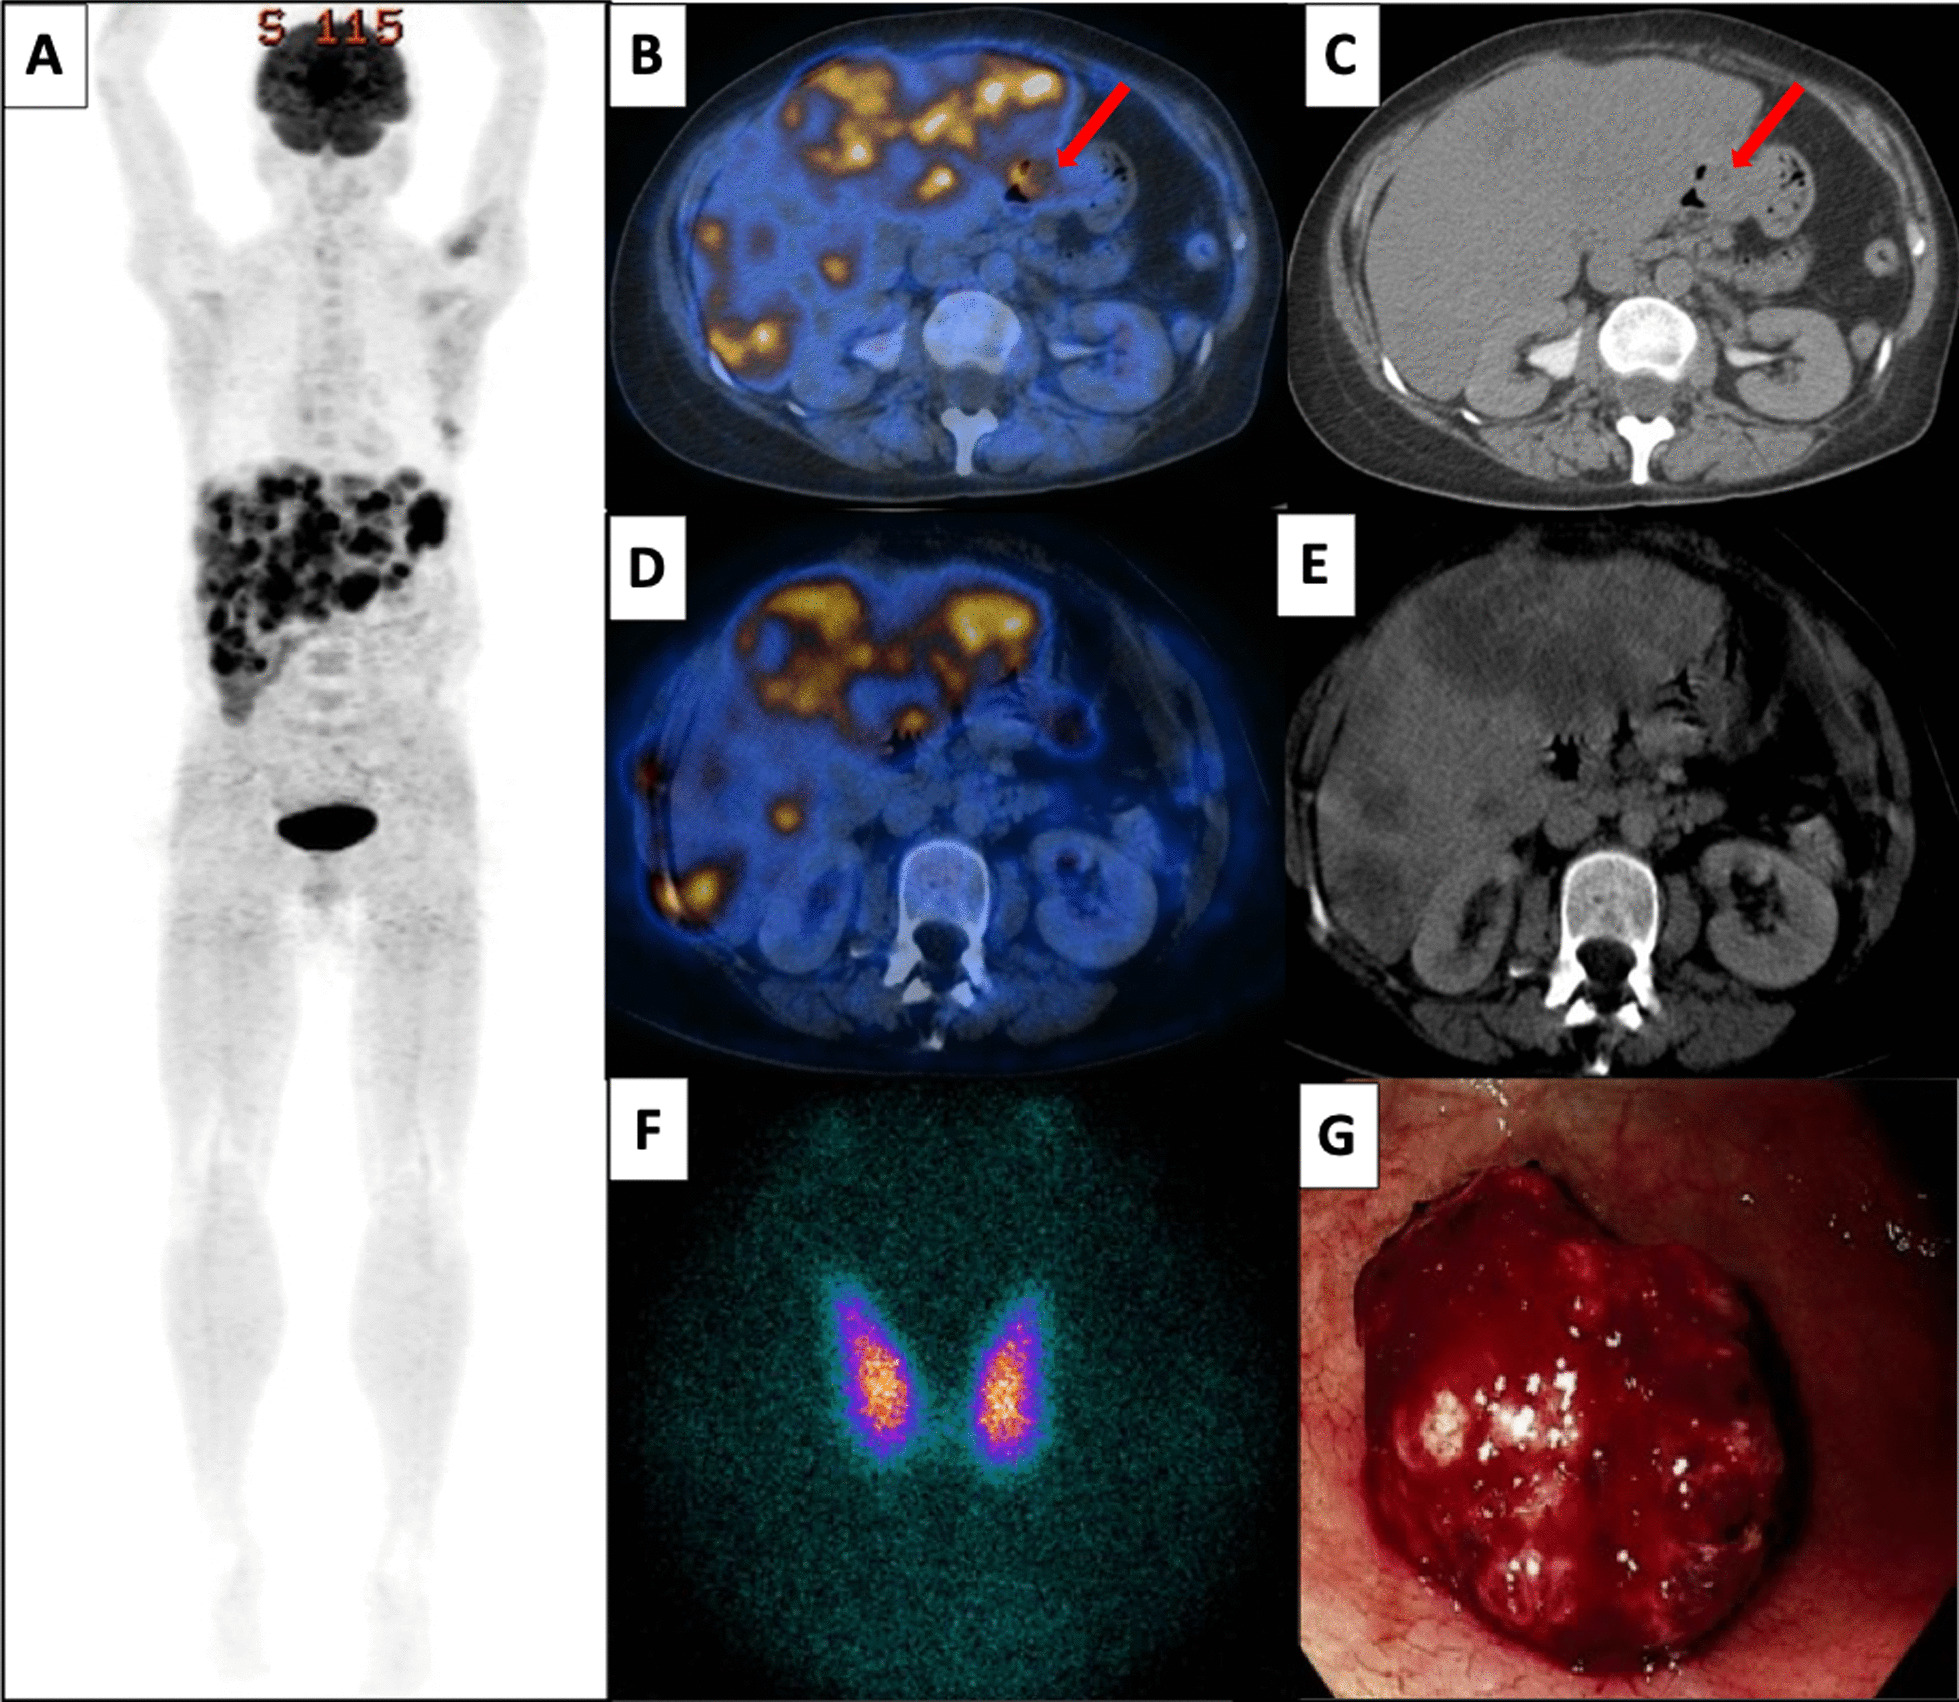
Imaging studies upon presentation and upper endoscopy. A–E Contrast-enhanced ^18^FDG PET-CT exhibited hepatomegaly (22 cm longitudinal axis) with multiple large FDG-avid liver masses, measuring up to 12 cm and SUV max of 13.5. A focus of FDG avidity in the gastric antrum had no structural correlate (red arrows), with an SUV max of 9.4. CT also showed mesenteric lymph nodes and small lung infiltrates. F Thyroid scan (^99m^TcO_4_^−^) with tendency to diffusely increased tracer uptake (radioiodine thyroid scan was not performed due to use of IV-iodinated contrast in the preceding two days). G Upper endoscopy showing a ~ 5 cm bleeding lesion in the greater stomach curvature body
